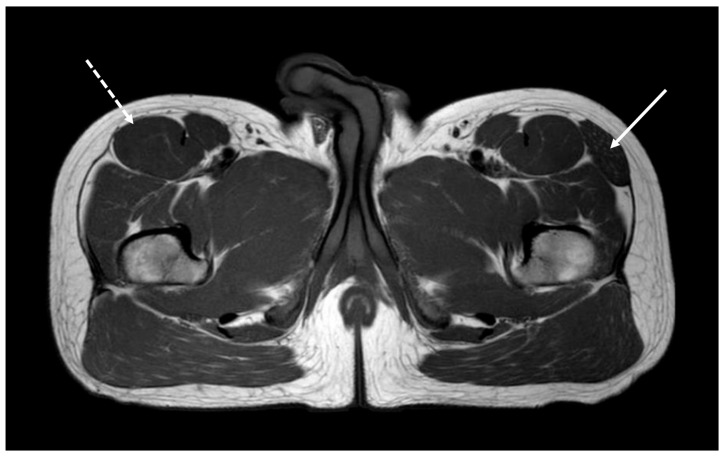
A 25-year-old physician born from non-consanguineous parents was included as a healthy control in a muscle-MRI study conducted by the third author (G.B.) on genetic myopathies as a part of his pediatric residency thesis. T1-weighted axial sections performed at the lower girdle level surprisingly showed the agenesis of the right TFL with mild compensatory hypertrophy of the ipsilateral rectus femoris (dashed arrow) and unaffected surrounding tissues. A normal appearance of the TFL was noted on the left side (arrow). Muscle MRI was bilaterally normal at thigh and leg levels. The tensor fasciae lata (TFL) is a muscle of the proximal anterolateral thigh that lies between the superficial and deep fibers of the iliotibial (IT) band [1]. There is high variability in muscle belly length, although, in most patients, the TFL muscle belly ends before the greater trochanter of the femur [1,2]. The exact role of TFL is not entirely clear. However, it cooperates with the gluteus maximus, gluteus medius, and gluteus minimus in various hip movements, including flexion, abduction, and internal rotation [1]. Furthermore, TFL acts via the IT band’s attachment to the tibia to assist with knee flexion and lateral rotation. The TFL can be considered clinically important for assisting pelvic stability while standing and walking [1]. Although rarely reported, conditions associated with TFL impairment may present as pain and dysfunction in the hip, pelvis, and spine region [3]. These conditions include benign and malignant lesions, such as lipoma, liposarcoma, intramuscular lymphoma and metastases, arteriovenous malformations, muscular atrophy, and adipose pseudohypertrophy [3]. Congenital agenesis of the TFL muscle is an extremely rare anomaly in which the muscle fails to develop during embryogenesis due to unknown reasons. Very little is known about the impact of TFL absence on human life [4,5].
